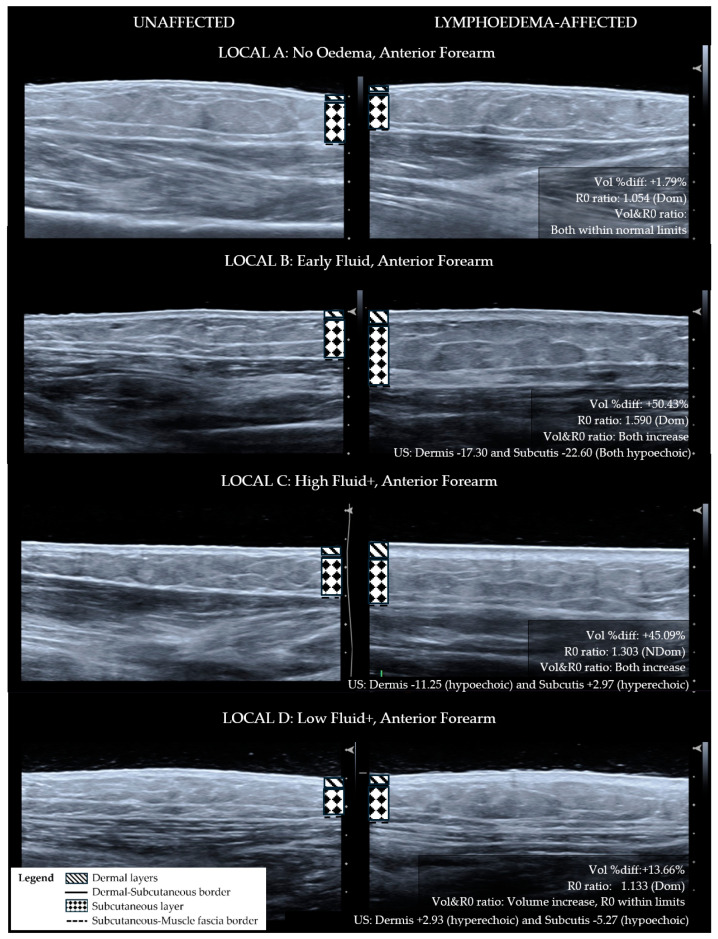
Ultrasound images for anterior forearm sites categorised with LOCAL criteria—matched lymphoedema-affected and unaffected sites. Lymphoedema-unaffected site (left), lymphoedema-affected test site (right). LOCAL A: No tissue characteristic change. LOCAL B: Lymphoedema dermal thickness increase and full-thickness hypo-echogenicity of the subcutis compared to the unaffected site. LOCAL C: Lymphoedema dermal thickness increase with hyperechoic change along the dermal–subcutaneous border and within the subcutis. LOCAL D: Lymphoedema dermal thickness increase with hyperechoic change along the dermal–subcutaneous border.
